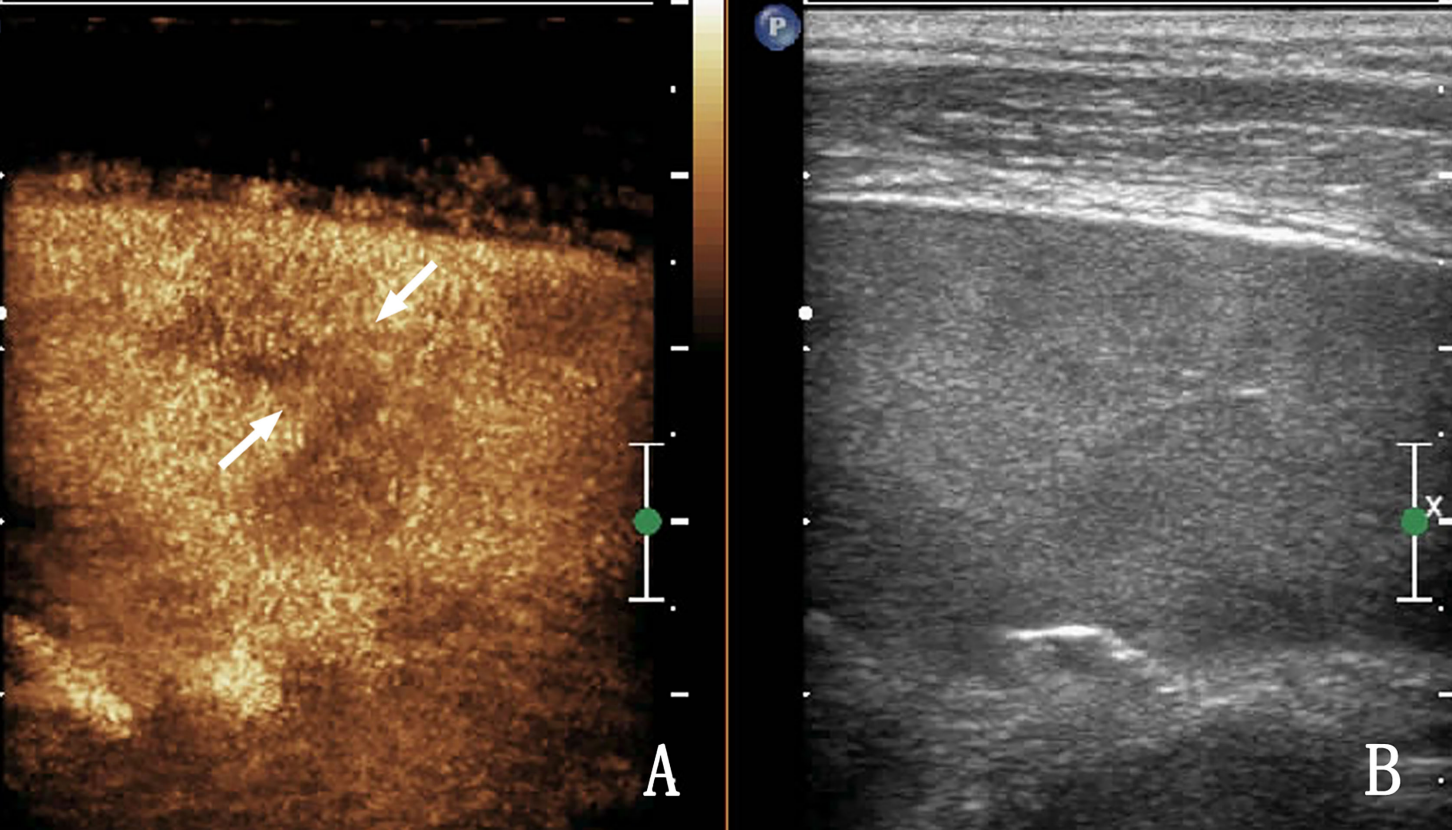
The same patient as that in Figure 2. (A) Contrast-enhanced ultrasound (CEUS) shows a low enhancement area in the spleen, and nodules appear to be conglomerated with each other (arrow) without obvious capsule enhancement. (B) Shows a two-dimensional ultrasound (2D US) image. Ultrasound-guided biopsy of the low enhancement area pathologically confirmed splenic lymphoma.
